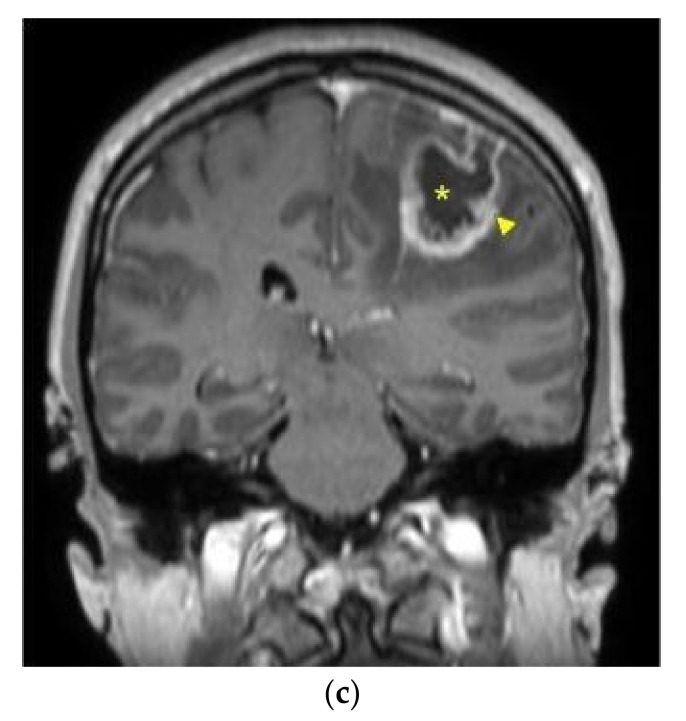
Brain MRI. A 3 cm left parietal solid mass (circle) surrounded by edema is demonstrated at brain MRI; its characteristics are typical for gliobastoma. Coronal FLAIR image (T2-weighted with CSF suppression; (a) and T2-weighted axial image (b) show hyperintense lesion (circle) with heterogeneous signal because of hemorragic intralesional foci and flow voids, surrounded by edema (arrowhead). In (b) both solid peripheral ring and necrotizing/hemorragic intralesional foci are better depicted. Coronal T1-weighted image after contrast (c) shows peripheral and irregular enhancement with nodular components (arrowhead), that surround necrosis (asterisk).
